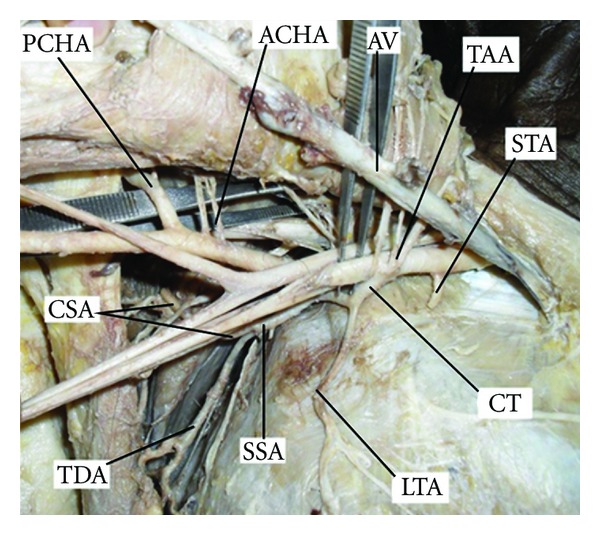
Dissection of right axilla shows the variant branching pattern of axillary artery. (AV: axillary vein, ACHA: anterior circumflex humeral artery, CT: common trunk, CSA: circumflex scapular artery, LTA: lateral thoracic artery, PCHA: posterior circumflex humeral artery, SSA: subscapular artery, STA: superior thoracic artery, TDA: thoracodorsal artery, and TAA: thoracoacromial artery).
